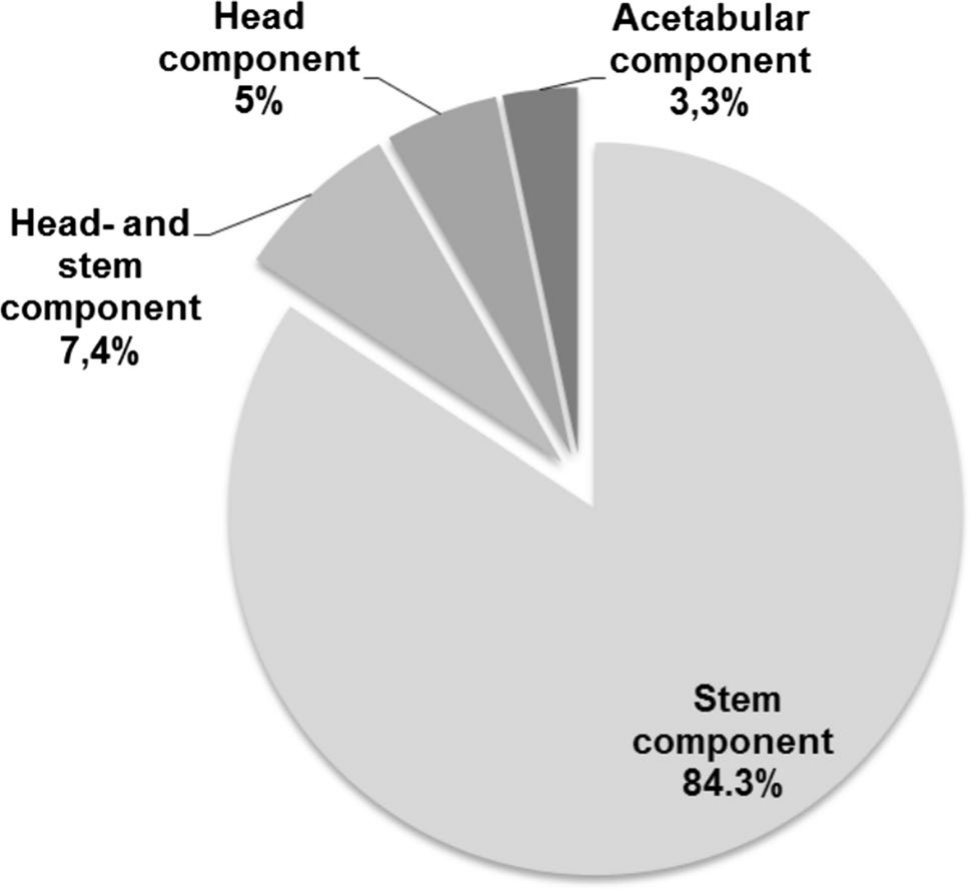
Percentage of intraoperative adjustments based on components after performing intraoperative radiography
